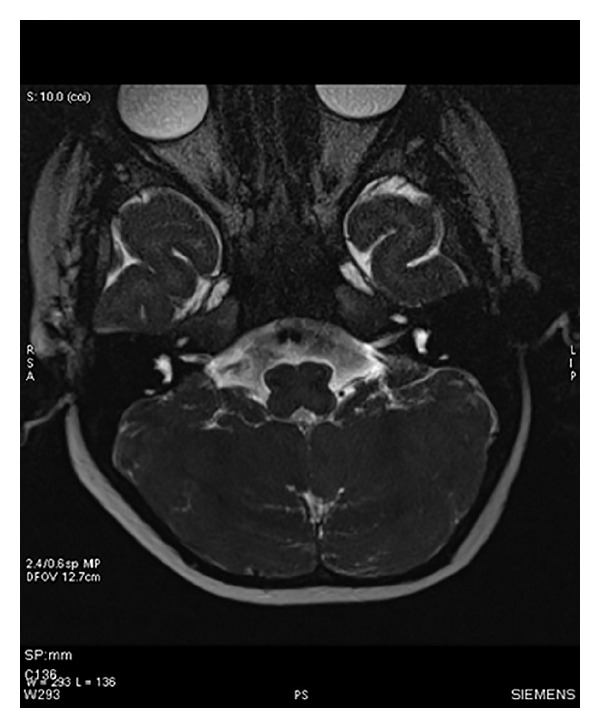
MRI scan of inner ear with axial section showing bilateral hypoplastic vestibulocochlear nerves.
